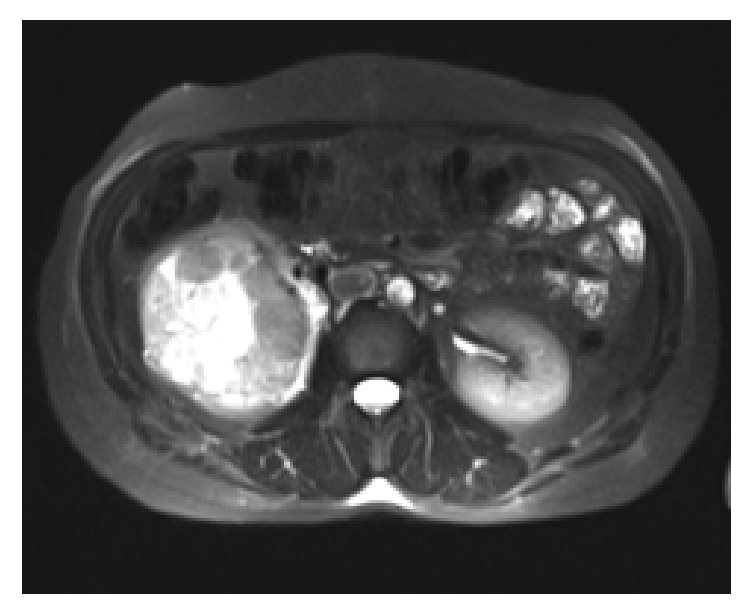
On contrasted CT, 80 mm × 76 mm renal mass with heterogeneous contrast enhancement arising from the lower pole of the right kidney.
